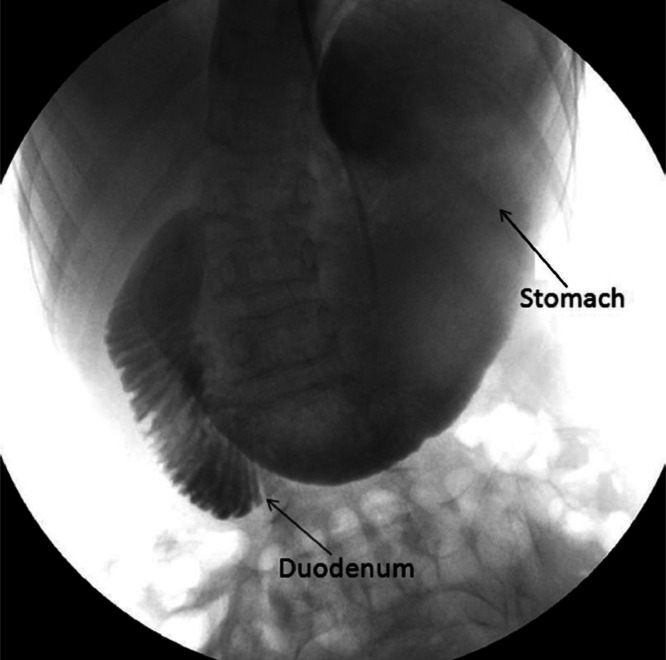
Gastroduodenal fluoroscopy. The fluoroscopy with iodinated contrast medium shows gastro-duodenal dilatation up to the third portion of the duodenum, which was previously found compressed by the superior mesenteric artery in CT with contrast medium. The last duodenal portion exhibits no contrast, suggesting that the contents’ passage is obstructed.
